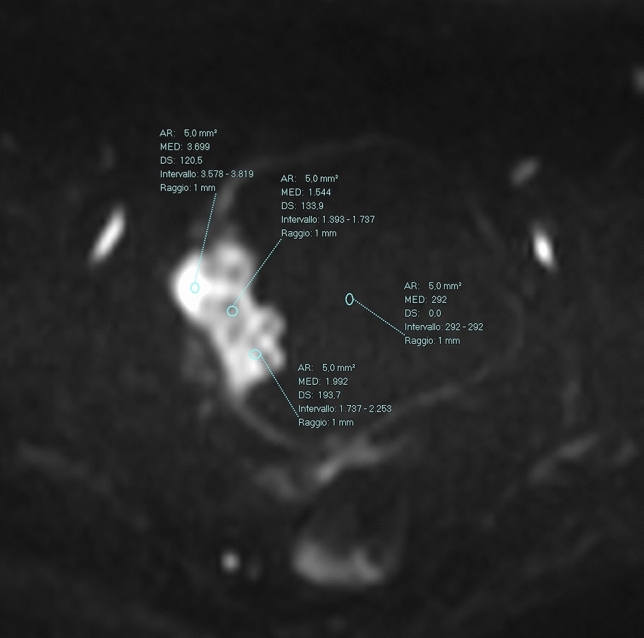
Quantitative analysis. Three circular 5-mm^2^ regions of interest (ROIs) of the tumor were placed on DWI images. Another circular 5-mm^2^ ROI was also placed in the bladder content to normalize data. Tumor conspicuity was defined as the ratio between the mean tumor intensity and the bladder content intensity
